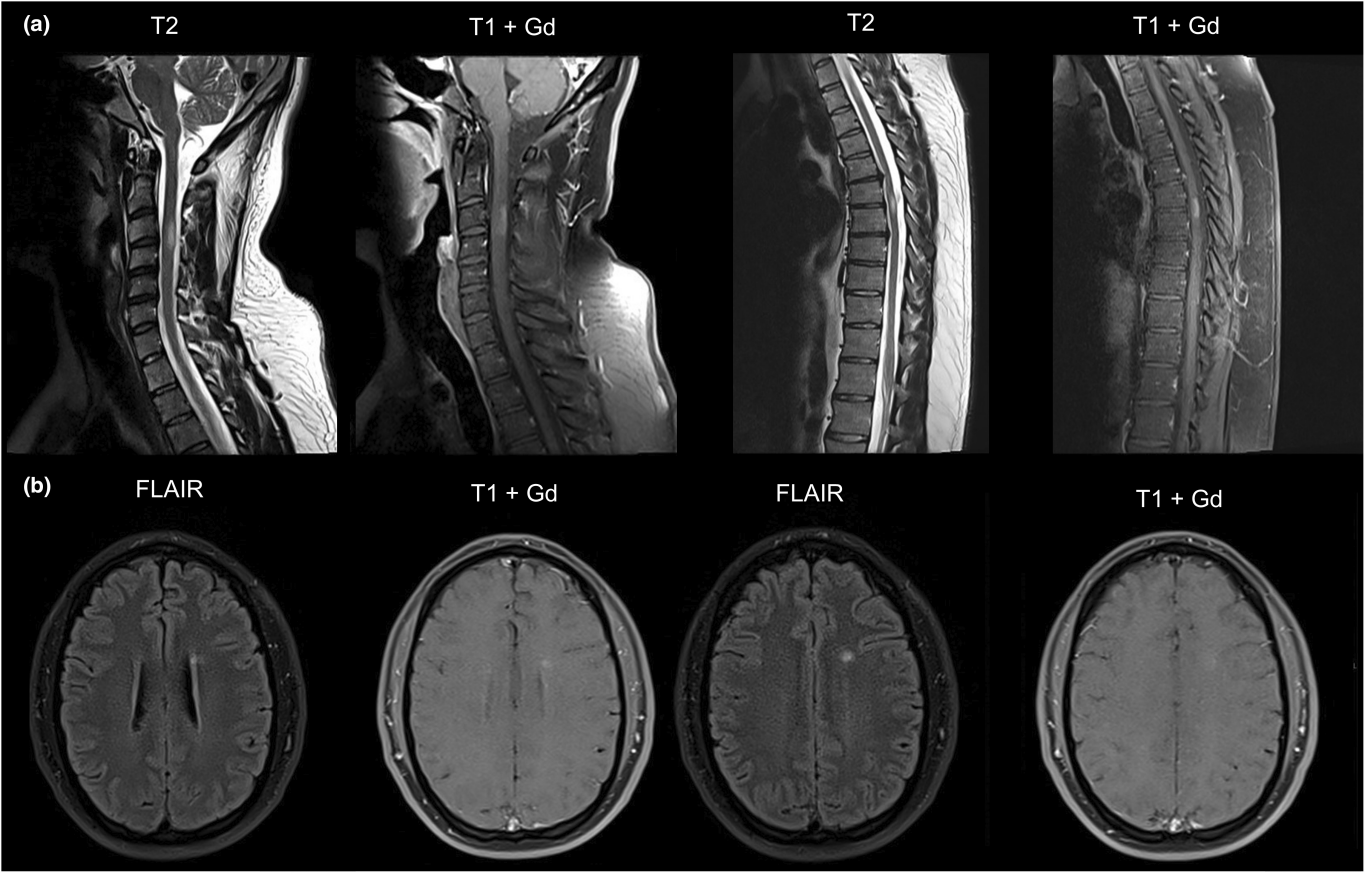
Magnetic resonance imaging of the spinal cord and the brain of the patient. (a) Hyperintense lesions are detected on both the cervical and the thoracic spine. (b) Periventricular lesion detected on the brain scans.
